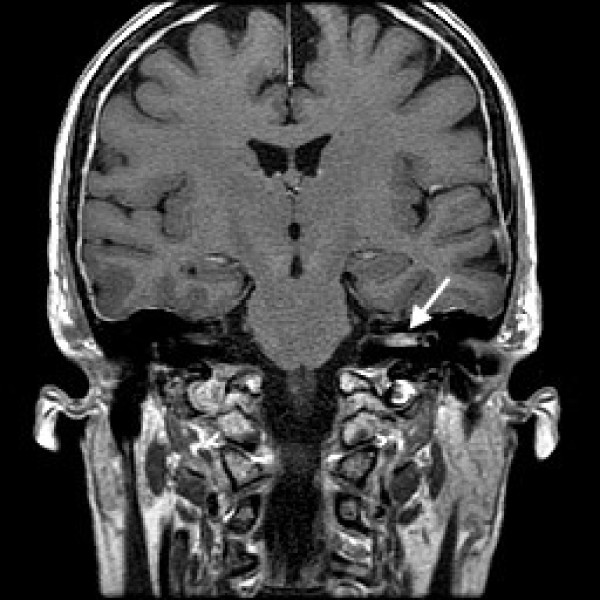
Coronal MRI image demonstrating the intracanalicular mass within the left internal auditory canal (arrowed) which enhanced with gadolinium.
